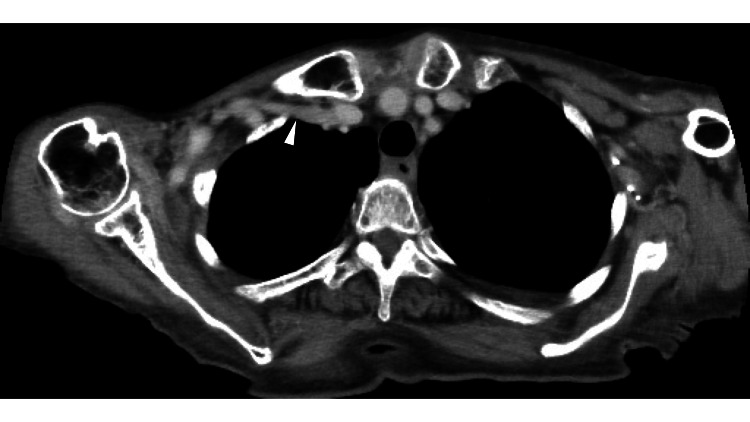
Computed tomography. Computed tomography in the normal position shows no significant stenosis of the right subclavian vein (white arrowhead).
